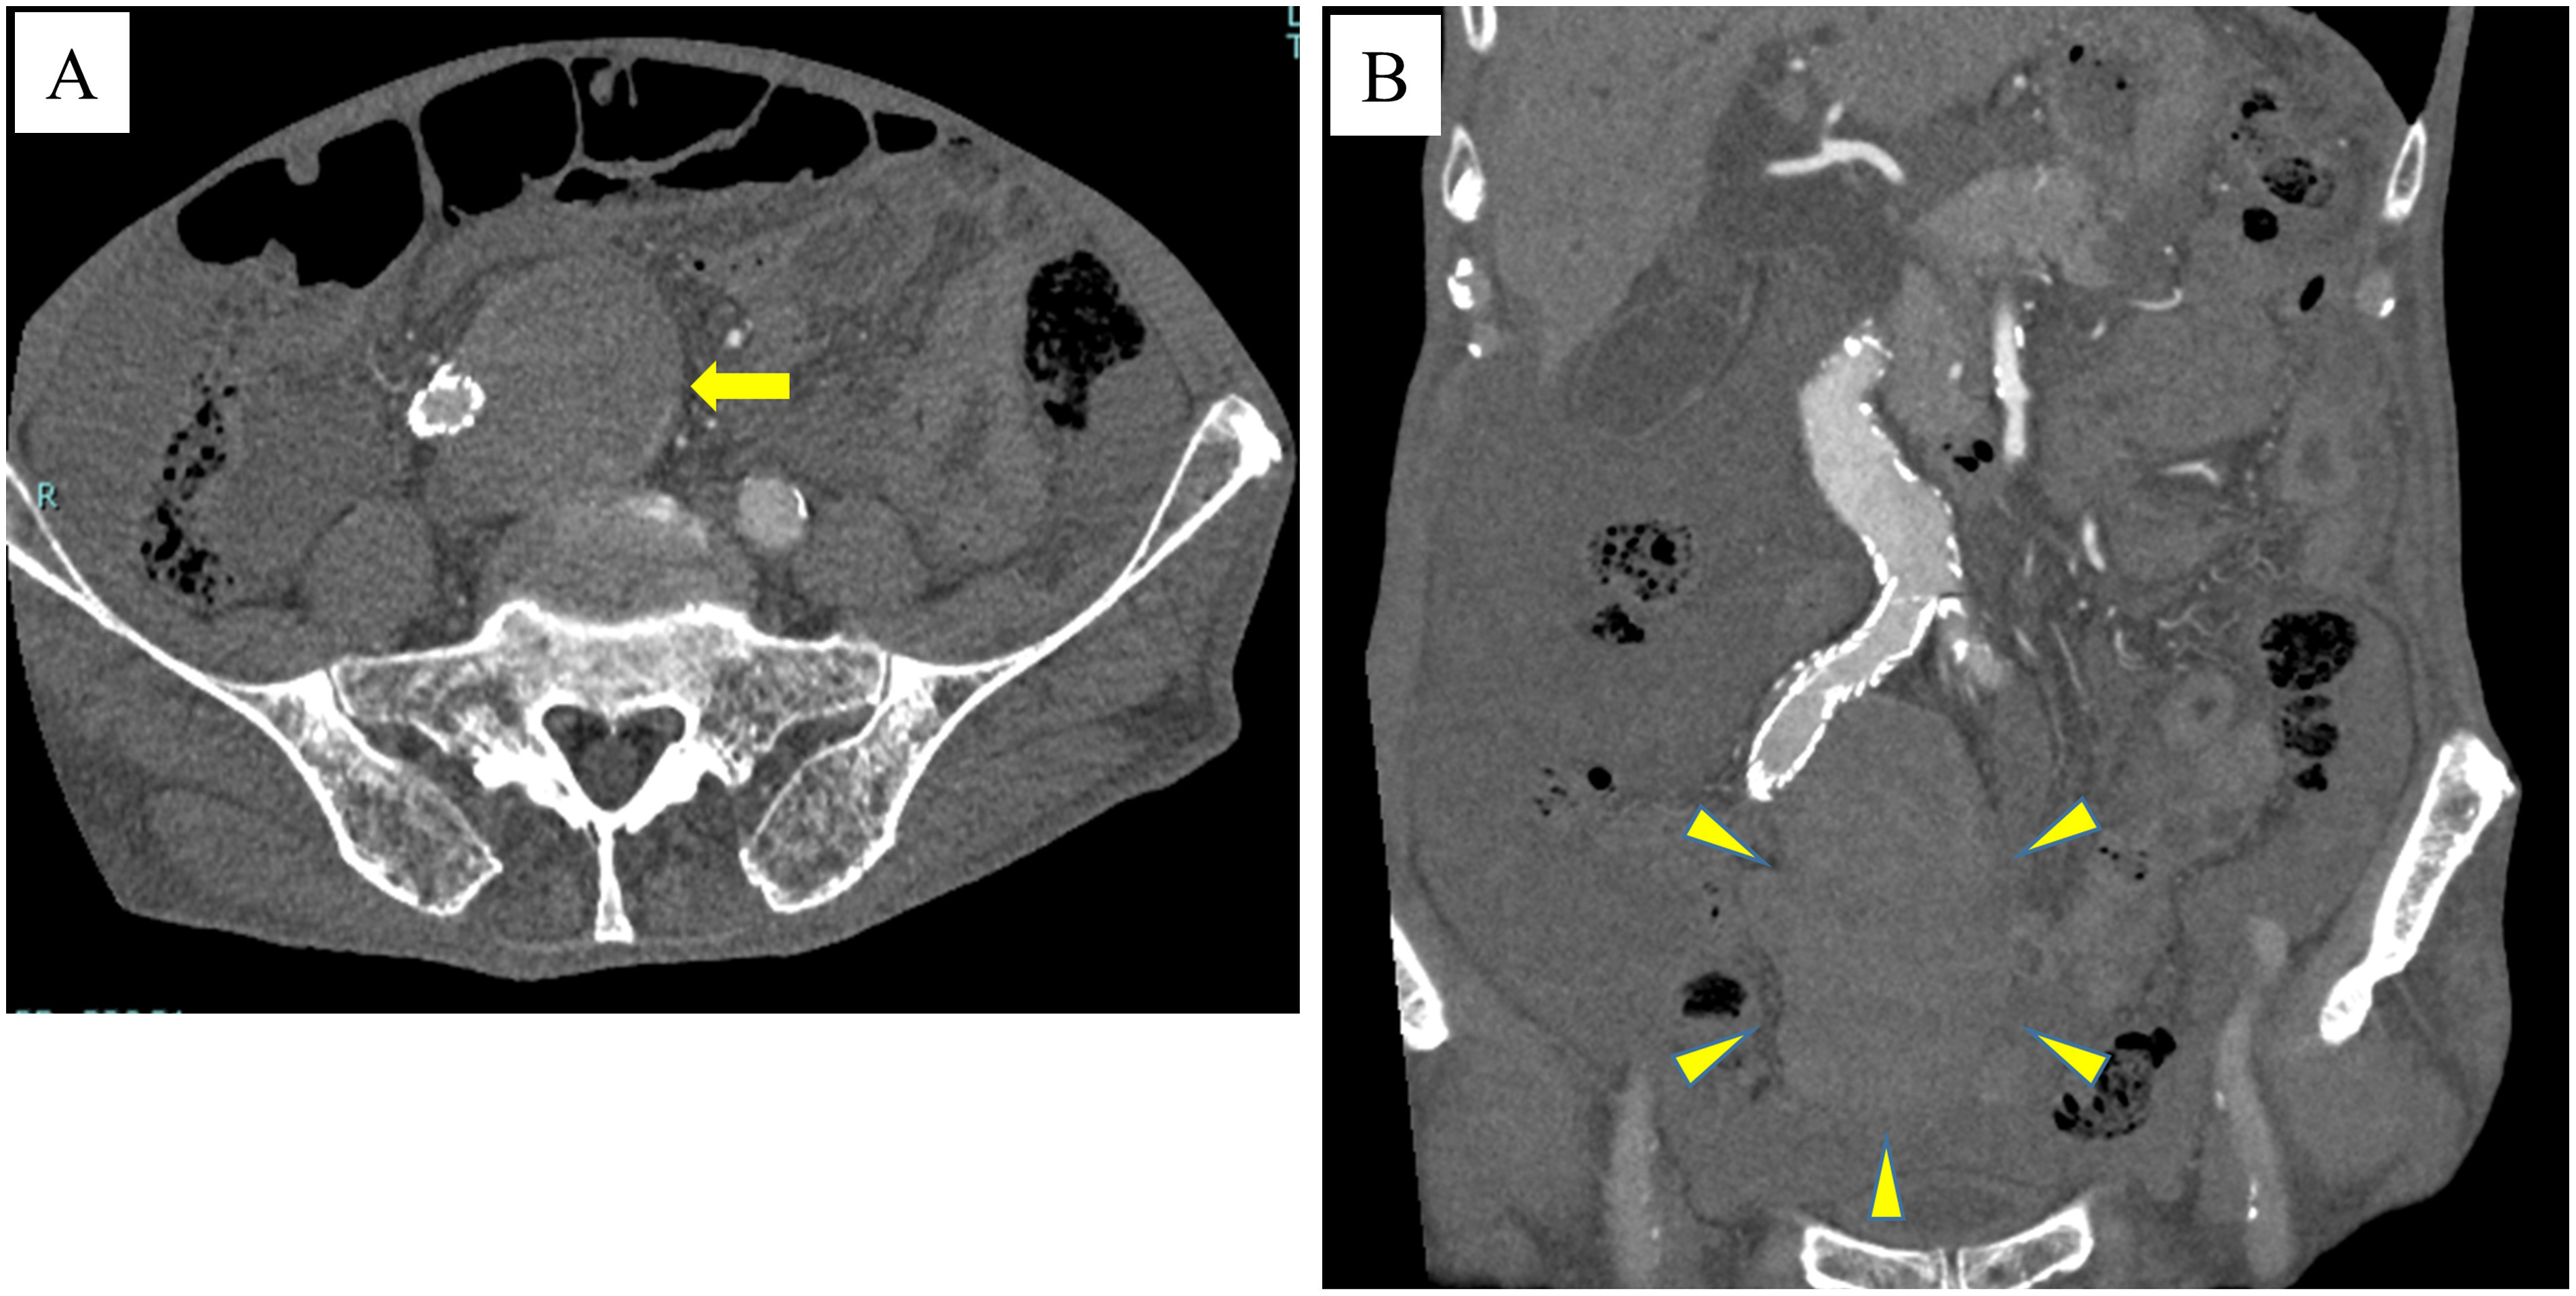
Fig. 3 (A), (B) Computed tomography showing a ruptured internal iliac artery aneurysm (arrow) and a large hematoma around the aneurysm (arrowheads).
